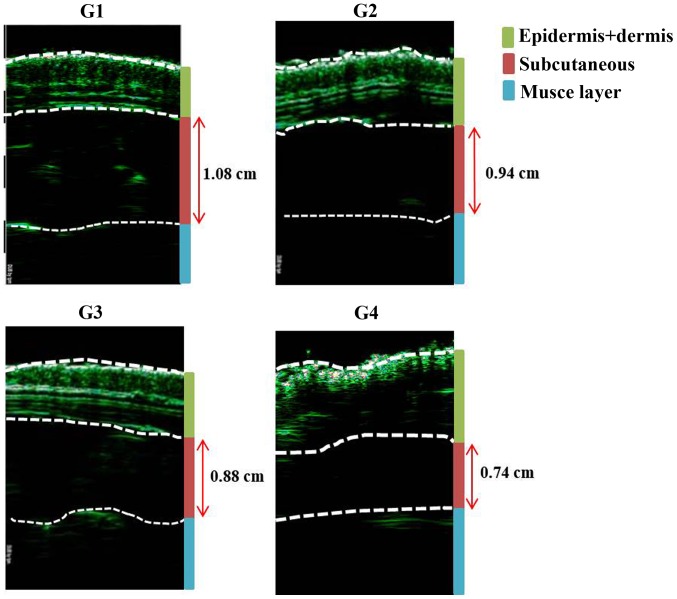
Comparison of fat layer thickness was performed using diagnostic ultrasound assessments on day 28. G1, untreated control; G2, topical cosmetic slimming cream alone without fabric; G3, slimming cream with normal fabric (made of 100% cotton); G4, slimming cream with the novel fabric.
